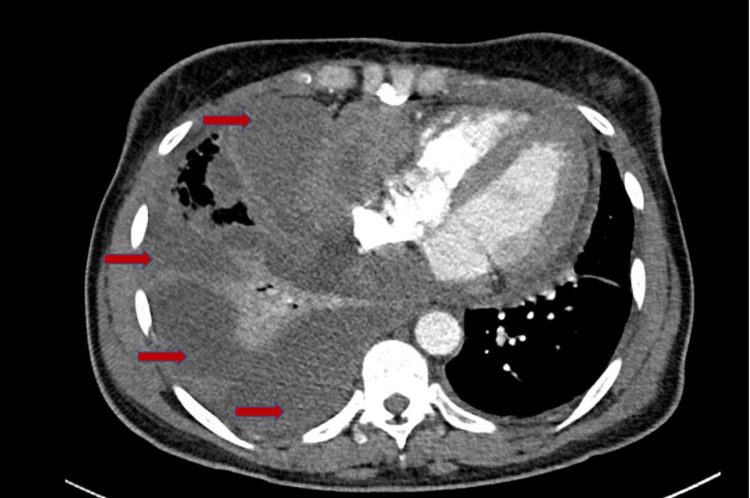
CT of chest showing multiple, large multi-loculated pleural effusions of the right hemithorax causing complete opacification (red arrows)
